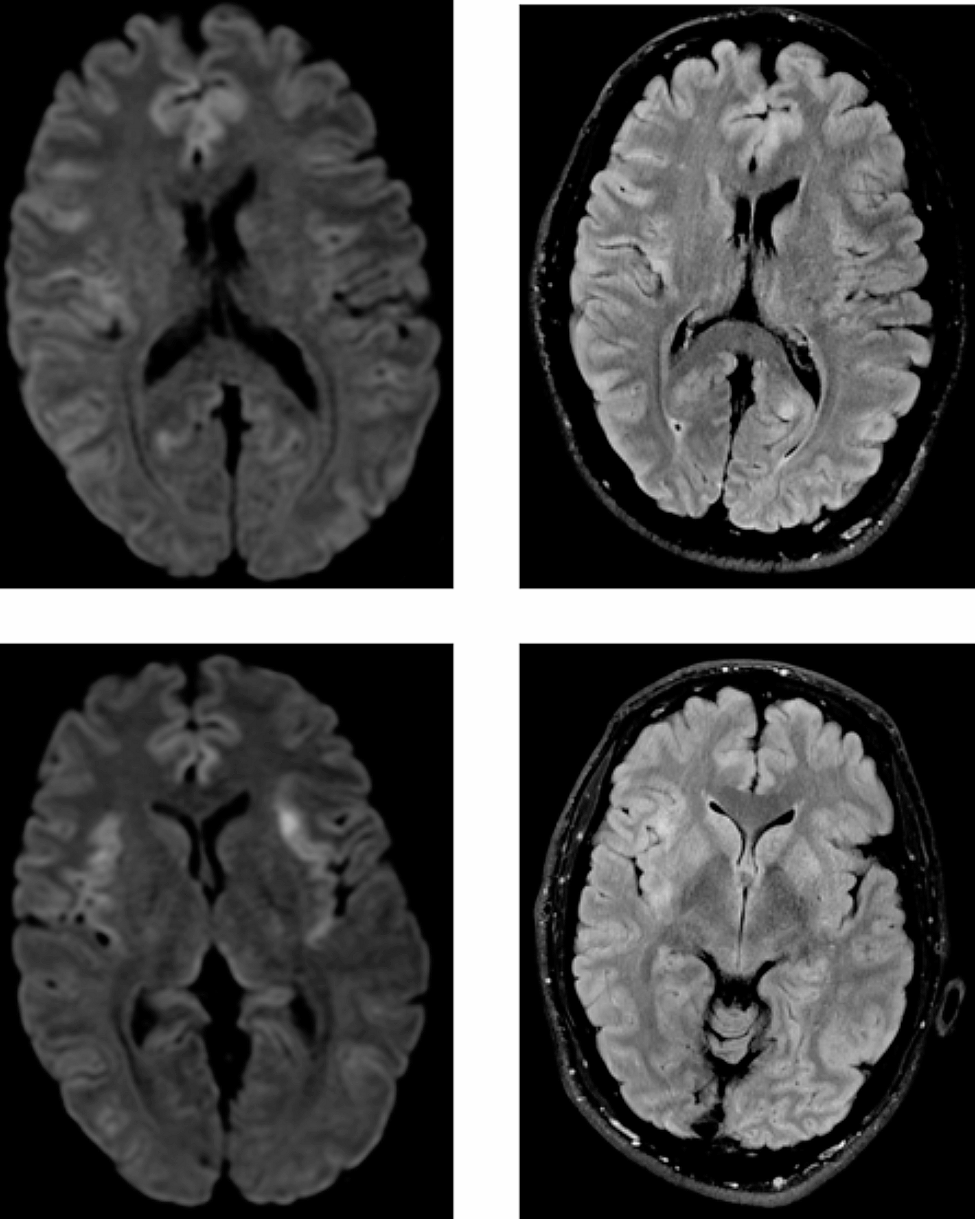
Magnetic resonance imaging (MRI) of the brain. MRI showed a high signal on diffusion weighted imaging in gyrus cinguli, insula and bilateral cortex. Due to agitation, some pictures hade movement artefacts and the MRI were disrupted before contrast injection
